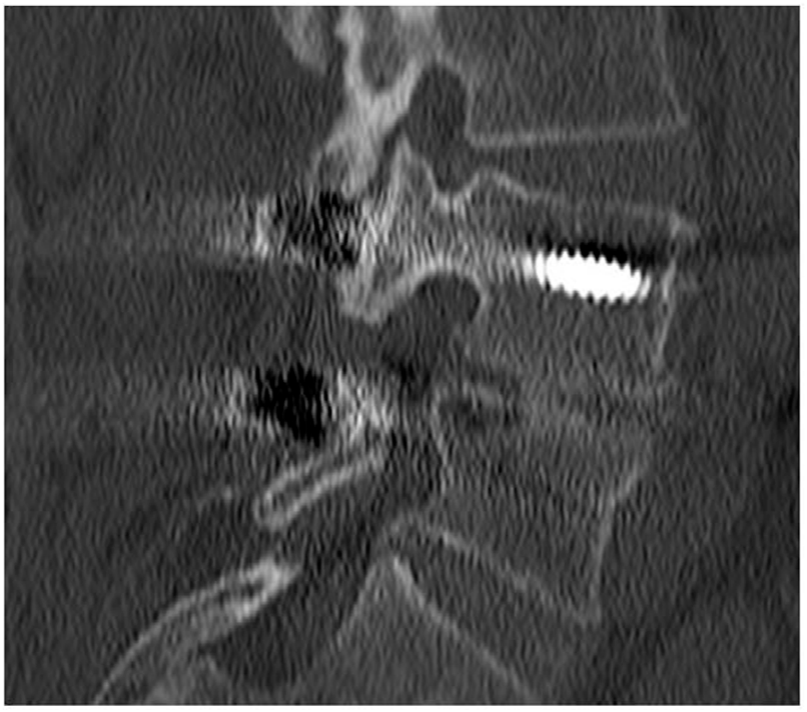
CT scan performed at 18-month follow-up, showing the absence of fusion (pseudoarthrosis) at levels L4–L5 and L5–S1 where SintLife was used.
